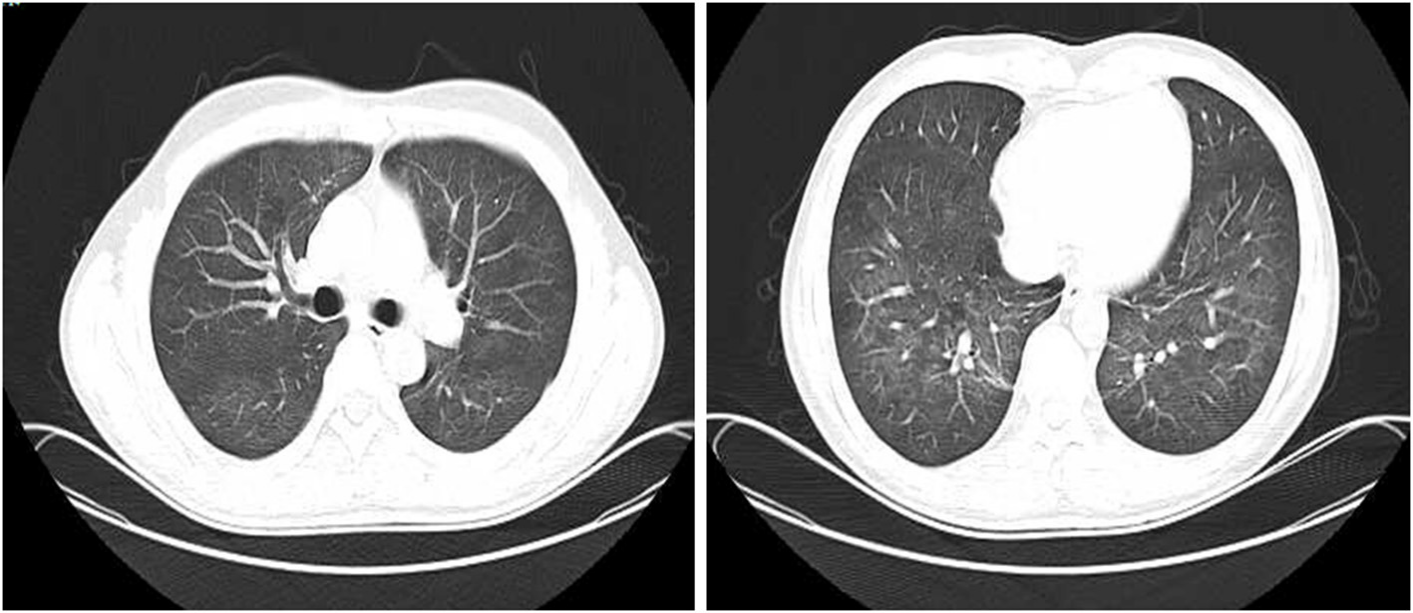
CT of the lung revealed multifocal bilateral ground-glass opacities on admission.
